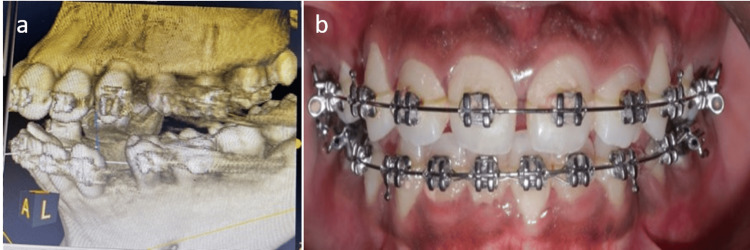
(a) Preoperative cone-beam computed tomography (CBCT) of the patient undergoing orthodontic treatment; (b) clinical preoperative view.
